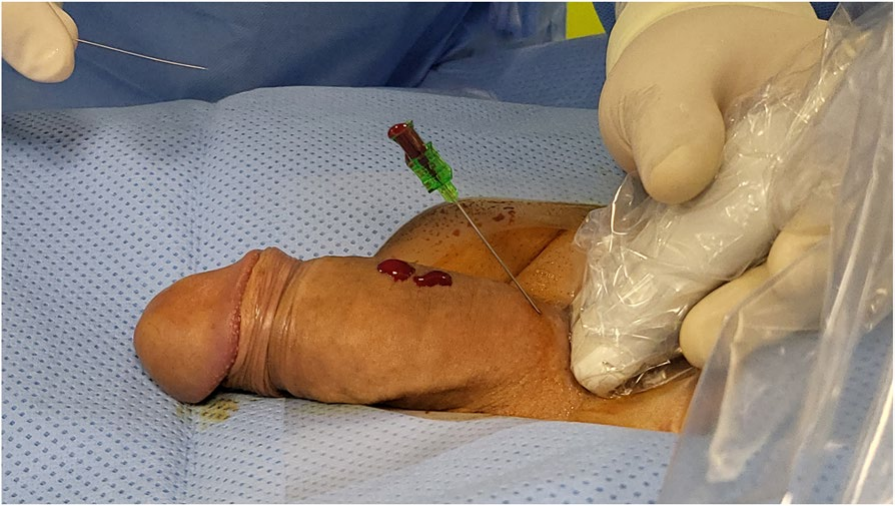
After subcutaneous administration of lidocaine 2% for local anesthesia and light sedation, ultrasound-guided puncture of a deep dorsal penile vein is performed using a 21-G micropuncture needle (Cook, U.S.A.). The needle is slowly advanced until blood returns from the needle hub (ref. corresponding movie file)
